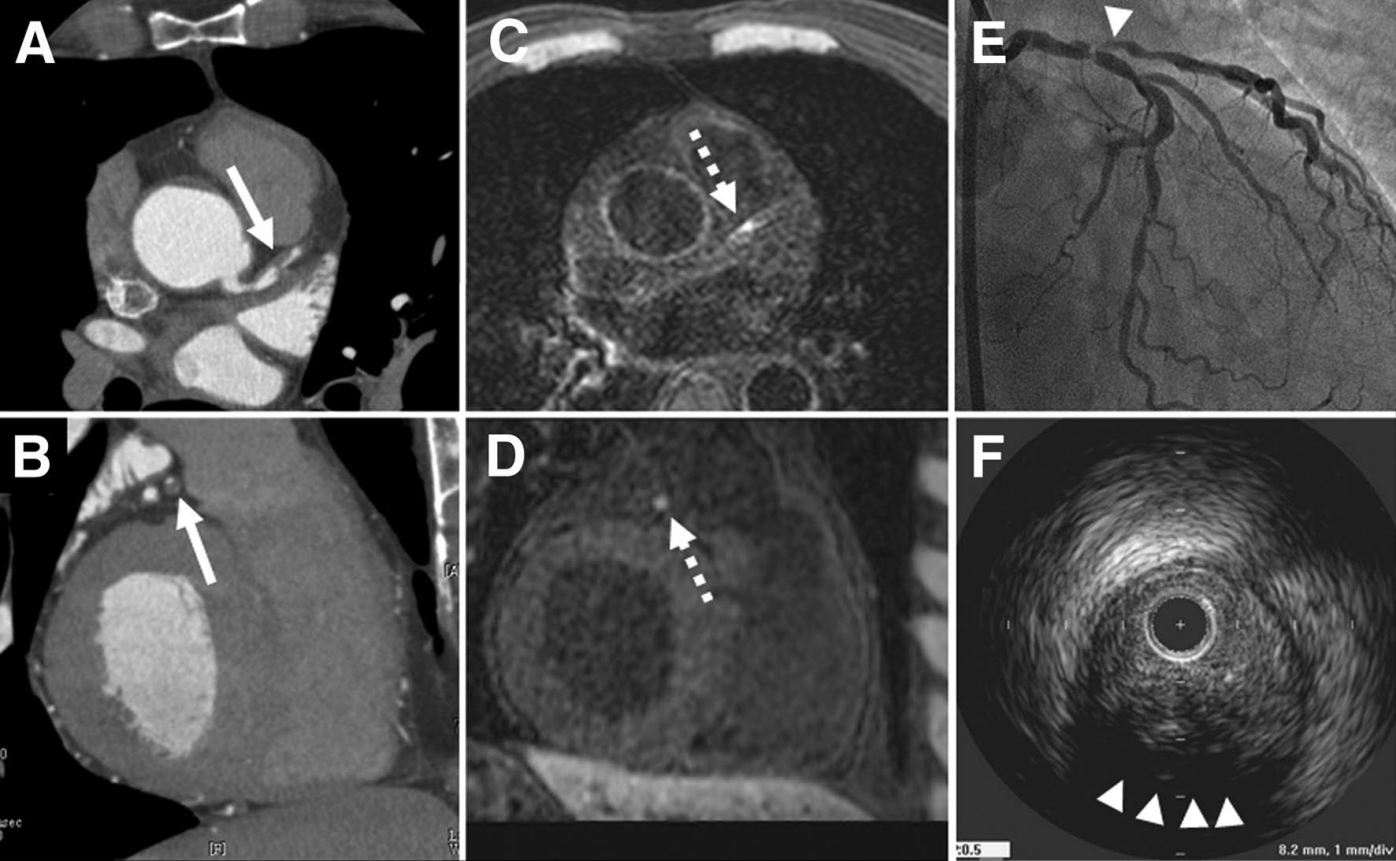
Representative case of HIP in the proximal LAD is presented. Coronary CTA (a horizontal, b sagittal) demonstrates the low-density positive remodeling plaque (− 32 Hounsfield units, remodeling index: 1.27) (arrow) with severe coronary stenosis in the proximal LAD. On the corresponding CMR (c horizontal, d sagittal), this low-density plaque was visualized as a “hyperintense spot” (dashed arrow). On the coronary angiography, severe coronary stenosis was observed (e) (arrowhead), and on IVUS examination (f), positive remodeling plaque (remodeling index: 1.29) with ultrasound attenuation (arrowheads) was observed in the proximal LAD, corresponding to the plaque observed by both coronary CTA and CMR. *HIP* hyperintense plaque, *LAD* left anterior descending artery, *CTA* computer tomography angiography, *CMR* cardiac magnetic resonance, *IVUS* intravascular ultrasound. (Reprinted with permission from Kawasaki et al. [121] Copyright © 2009 by the American College of Cardiology Foundation)
